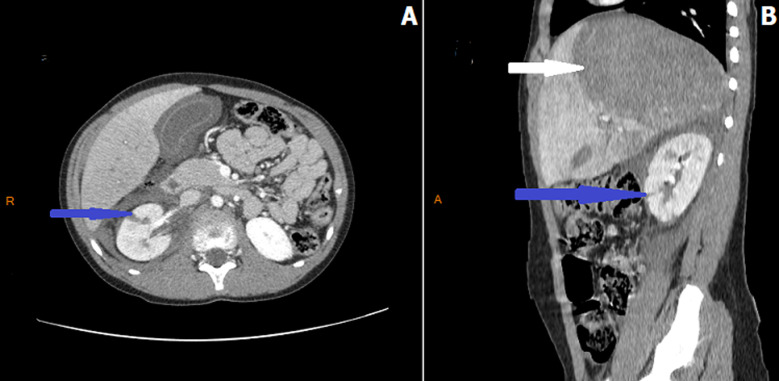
A) axial and B) sagittal abdominal contrast enhanced CT performed after a blunt abdominal trauma in an 11-years-old boy showing a parenchymal laceration of the right kidney (grade III of AAST Kidney Injury Scale) (blue arrows) associated with a voluminous hematoma of the liver (white arrow) after falling from standing height and who was managed conservatively
